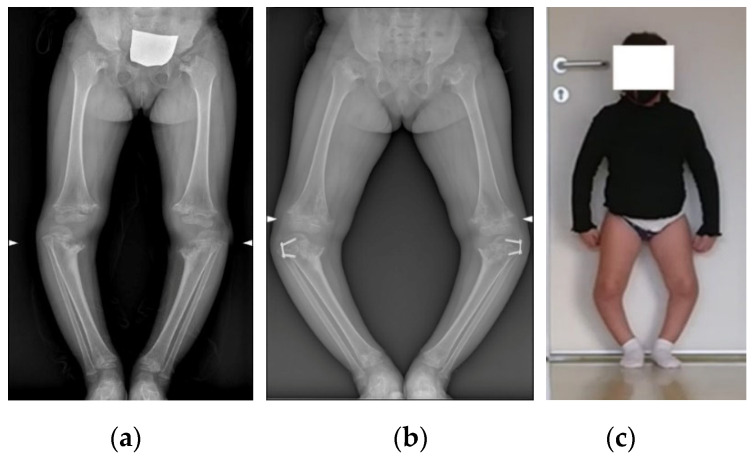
Preoperative longstanding radiographs of the patient (a) at the age of 4 years before tibial hemiepiphysiodesis with TBPs, (b) at the age of 7 years, and (c) clinical presentation at the age of 7 years.
